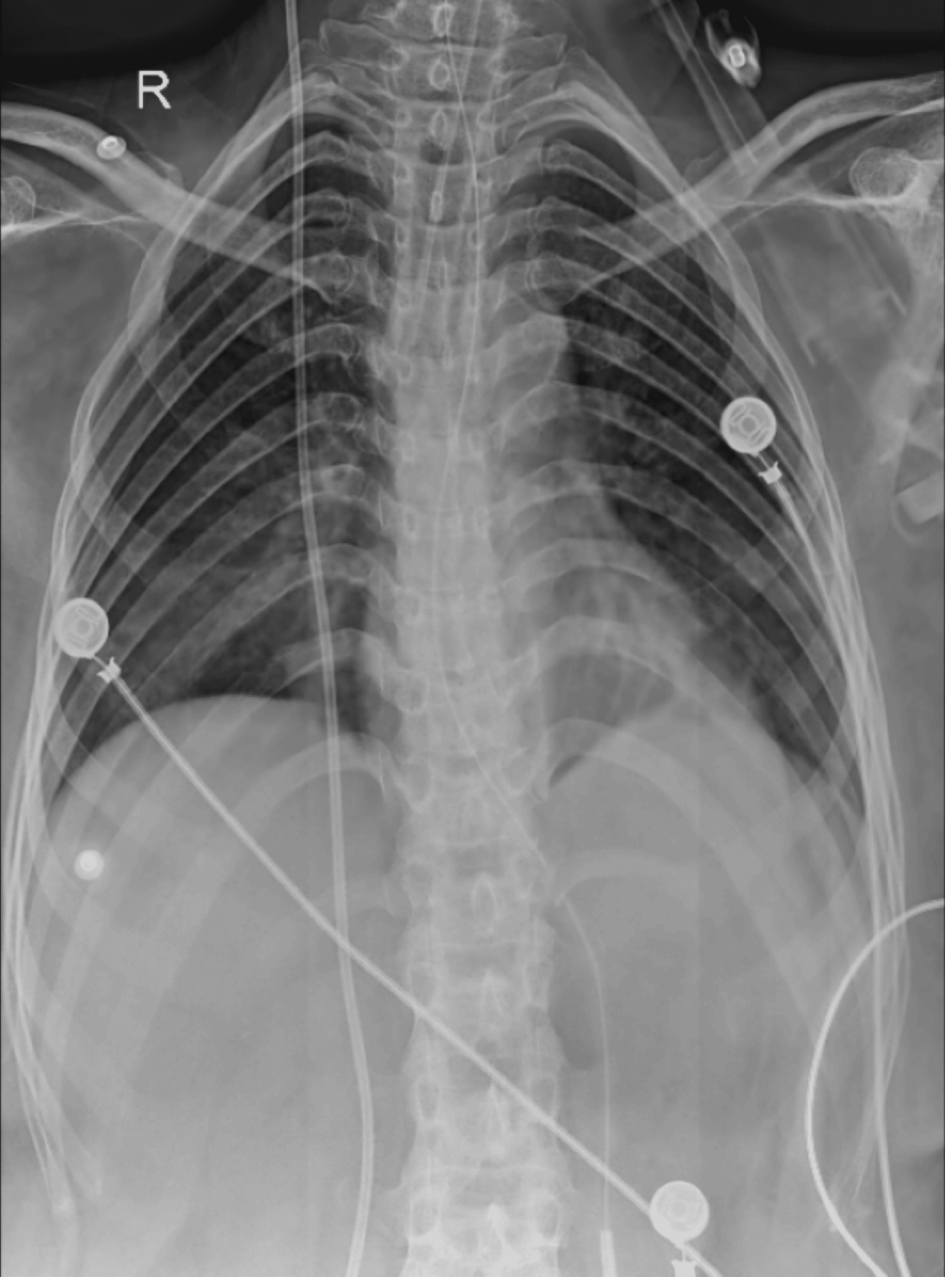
Chest X-ray showing the bronchovesicular markings present in left upper, middle, and lower zones
